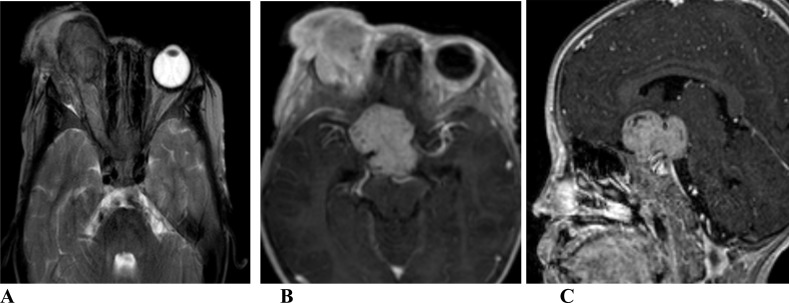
MRI of a 4 years-old female patient presenting with right eye swelling of 2 month duration. There is right intraocular ill-defined mass replacing most of the globe with proptosis. The mass has intermediate signal on T2 W (A) with intense contrast enhancement (B). There are similar signal intensity change following the right optic nerve and optic chiasm forming a suprasellar mass (B, C).
